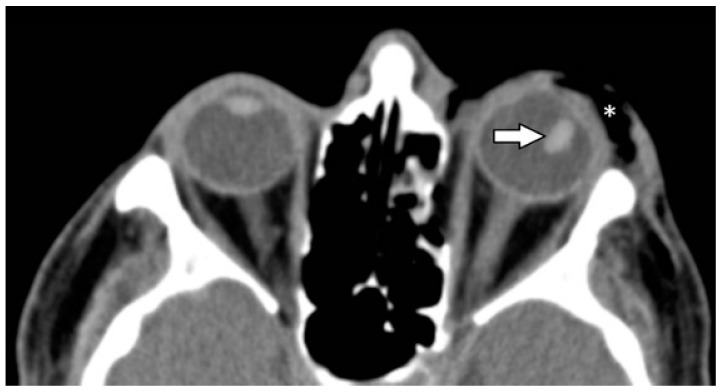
Dislocated left lens (indicated by arrow) and lateral rotation of about 70 degrees. Asterisk (*) indicates periorbital soft tissue emphysema due to a concomitant orbital floor fracture. NE-MSCT, soft tissue window, axial reformation (NE-MSCT = non-contrast-enhanced multi-slice CT).
